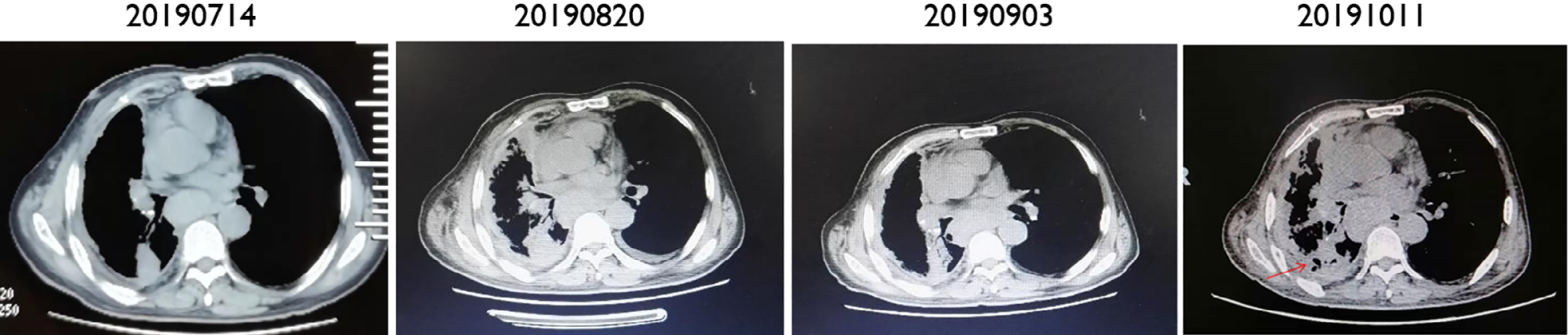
The CT scan during the treatment. “20190714” were the imaging findings 6 months after afatinib (before osimertinib); “20190820” were the imaging findings 1 month after osimertinib (before osimertinib + afatinib); “20190903” and “20191011” were the imaging findings 1 month and 2 months after osimertinib + afatinib.
